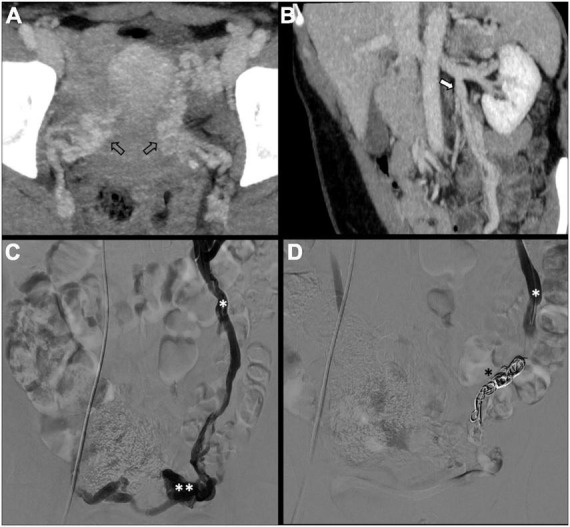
Type Ia pelvic varices in a 48 yo female due to unilateral venous insufficiency. (A,B) Computed tomography (CT) phlebography shows pelvic varices (open arrows) with a dilated left ovarian vein (white arrow). (C,D) Conventional phlebography and CT phlebography show pelvic varices (double white asterisks) with a dilated left ovarian vein (single white asterisk), treated with multiple coils (single black asterisk).
